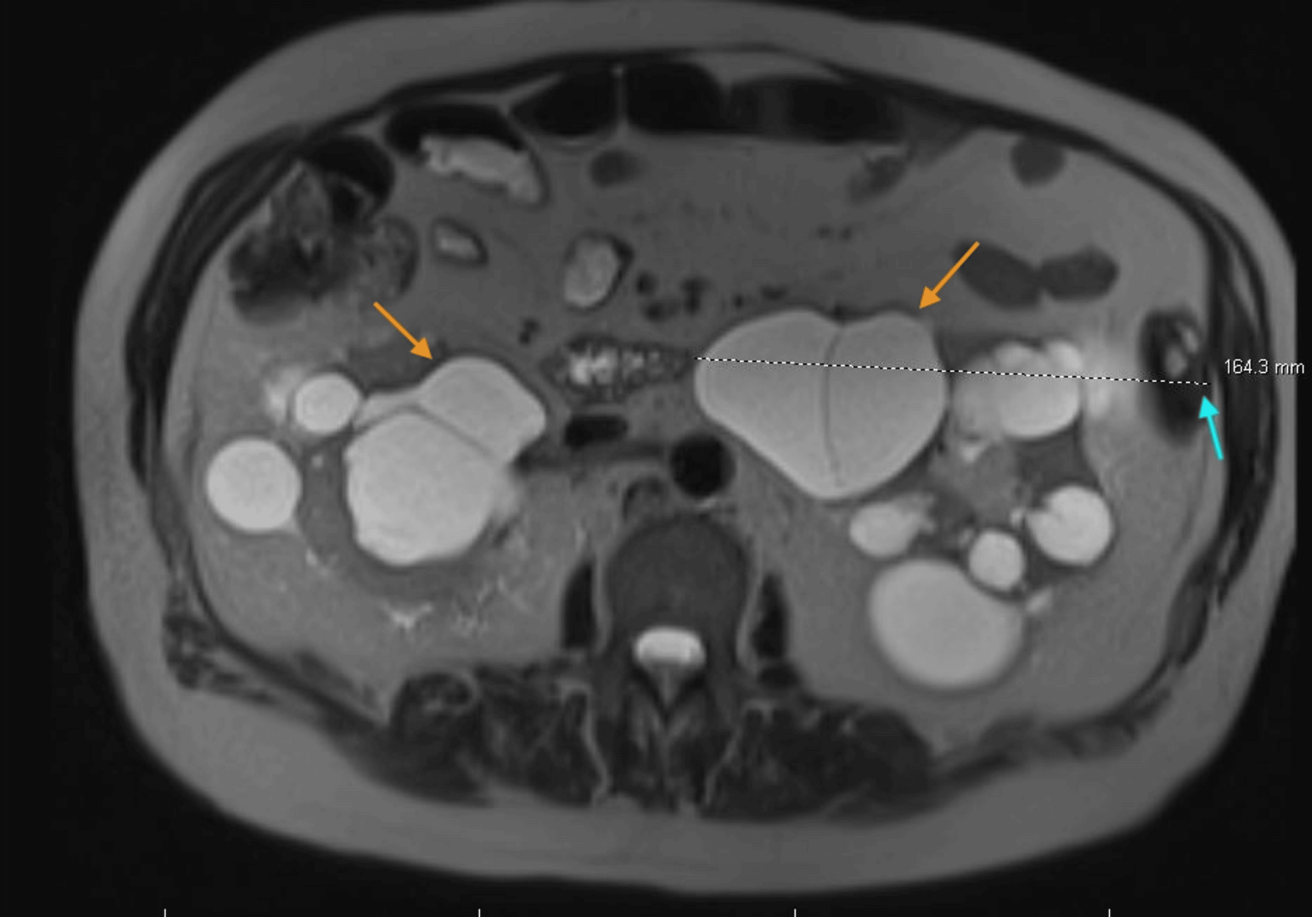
MRI images showing bilateral enlarged kidneys with multiple renal cysts Orange arrows indicate renal cysts. The blue arrow indicates the left kidney width measurement. MRI: magnetic resonance imaging
